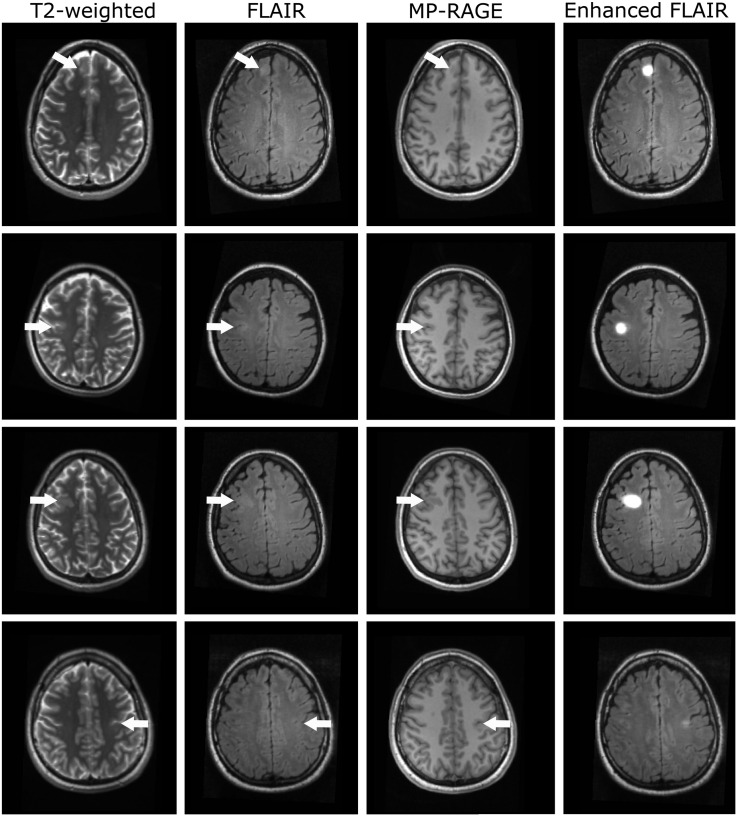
Representative datasets of four patients with FCD (rows). From left to right: T2-weighted, FLAIR-, MP-RAGE- and enhanced FLAIR-datasets. Arrows indicate the locations of the FCDs.
